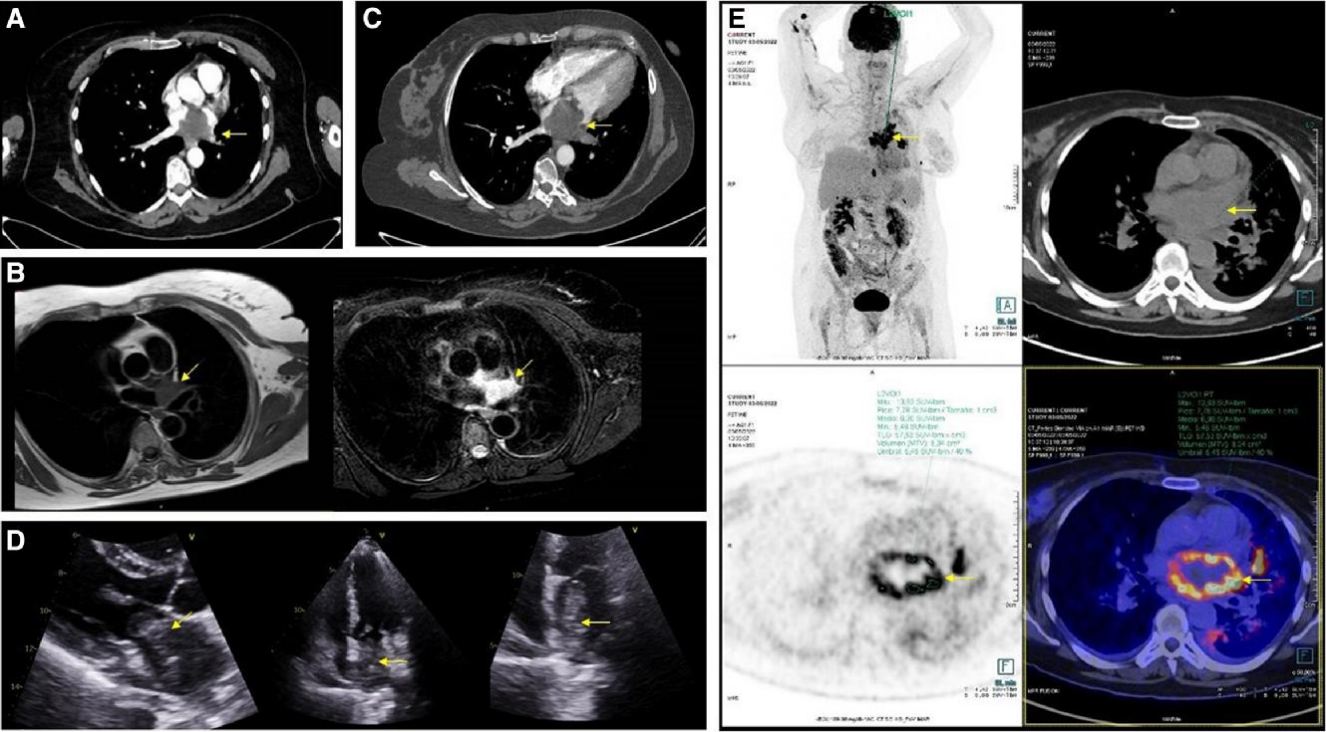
(*A*) Initial computed tomography angiography showing a mass within the left atrium suggestive of either a thrombus or a tumour lesion. (*B*) Cardiovascular magnetic resonance showing a heterogeneous image, isointense on T1-weighted images and hyperintense on T2-weighted image, with no late gadolinium enhancement, which protruded into the left atrium from the outlet of the left upper pulmonary vein. (*C*) Second computed tomography angiography showing a growth of the mass size, which occupies almost the entire left atrium. (*D*) Transthoracic echocardiogram showing a 55 × 45 mm non-pedunculated multilobulated motionless mass of inhomogeneous echogenicity attached to the atrial posterior wall extending into the PVs and the mitral valve. (*E*) Body FDG PET/computed tomography angiography showing images compatible with metastatic implants in lungs, bones, and muscles and left atrium mass uptake. PV, pulmonary vein; FDG, fluorodeoxyglucose; PET, positron emission tomography.
